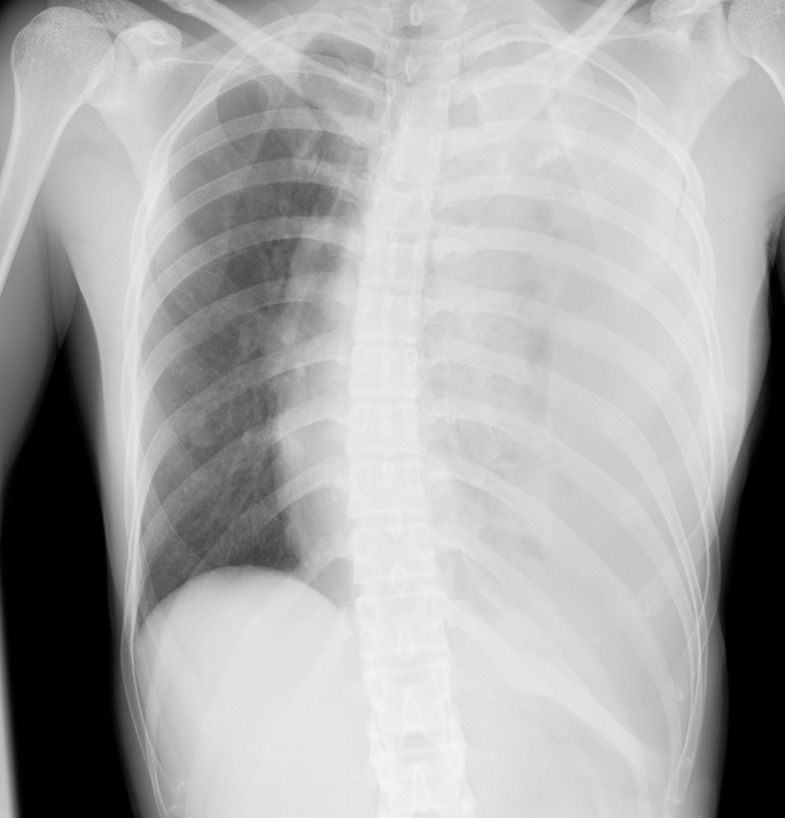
Chest radiograph on admission. Chest radiograph showing a massive left pleural effusion, with mediastinal deviation to the right.
